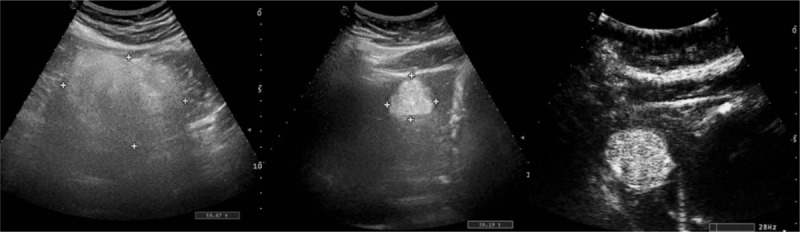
The contrast-enhanced ultrasound examination, which displayed that the blood supply of the left kidney mass is rich and the enhancement is uneven, presenting a “fast-forward and fast-out” mode.
